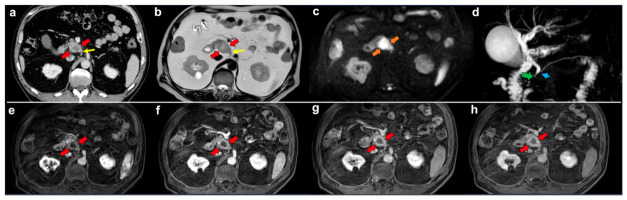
PDAC involving the uncinate process of the pancreatic head, in a male patient with painless jaundice, elevated bilirubin and increased CA 19-9. (a) Axial portal-phase CT demonstrates a hypovascular mass with a hyperenhancing rim in the uncinate process (red arrows), with focal infiltration of the adjacent retroperitoneal fat (yellow arrow). (b) Axial T2WI shows slightly heterogeneous hyperintensity of the mass (red arrows) and confirms retroperitoneal fat infiltration (yellow arrow). (c) DWI (b = 800 s/mm^2^) shows marked restricted diffusion within the pancreatic lesion (orange arrows). (d) Coronal 3D MRCP with MIP reconstruction demonstrates stenosis of the distal CBD (green arrow) with moderate upstream biliary dilatation, and focal stricture of the MPD (blue arrowhead) with minimal, regular upstream dilatation. (e–h) Dynamic contrast-enhanced MRI in the pancreatic (e), portal (f) and delayed phases acquired 3 and 5 min after contrast administration (g,h) confirms the presence of a persistently hypovascular mass with a hyperenhancing rim in the uncinate process (red arrows). In this case, the absence of homogeneous delayed enhancement and the presence of infiltrative margins were key findings favouring PDAC over focal AIP. The definitive diagnosis of PDAC was confirmed by EUS-FNA.
